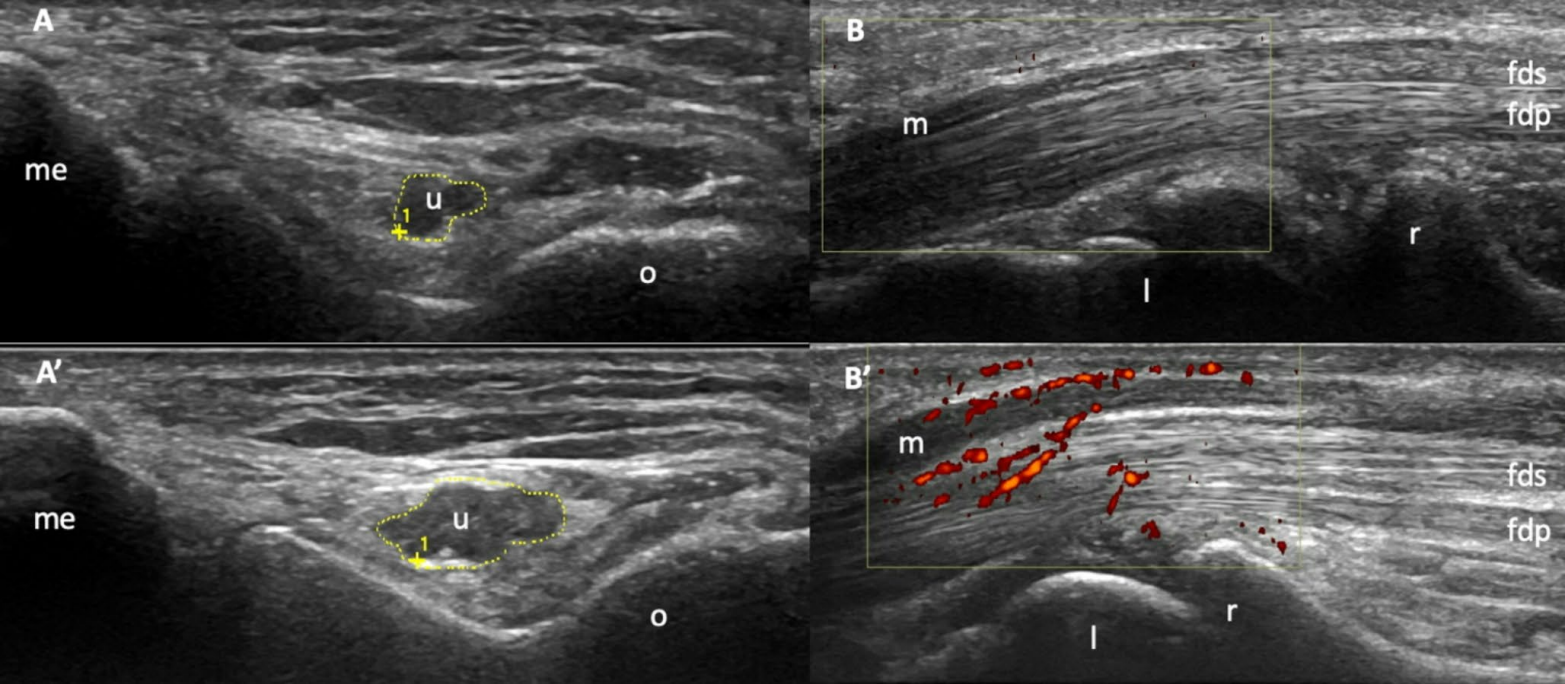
Ultrasound in the assessment of nerves (compressive neuropathty). A shows a transverse view of a normal ulnar nerve at the elbow in a healthy subject, while A’ displays an enlarged ulnar nerve (increased cross-sectional area) in a patient with cubital tunnel syndrome. B presents a longitudinal view of a normal median nerve at the wrist in a healthy subject, and B’ shows an enlarged median nerve with power Doppler signal (red spots) in a patient with carpal tunnel syndrome. *fds* = flexor digitorum superficialis; *fdp* = flexor digitorum profundus; *l* = lunate; *m* = median nerve; *me* = medial epicondyle; *o* = olecranon; *r* = radius; *u* = ulnar nerve
